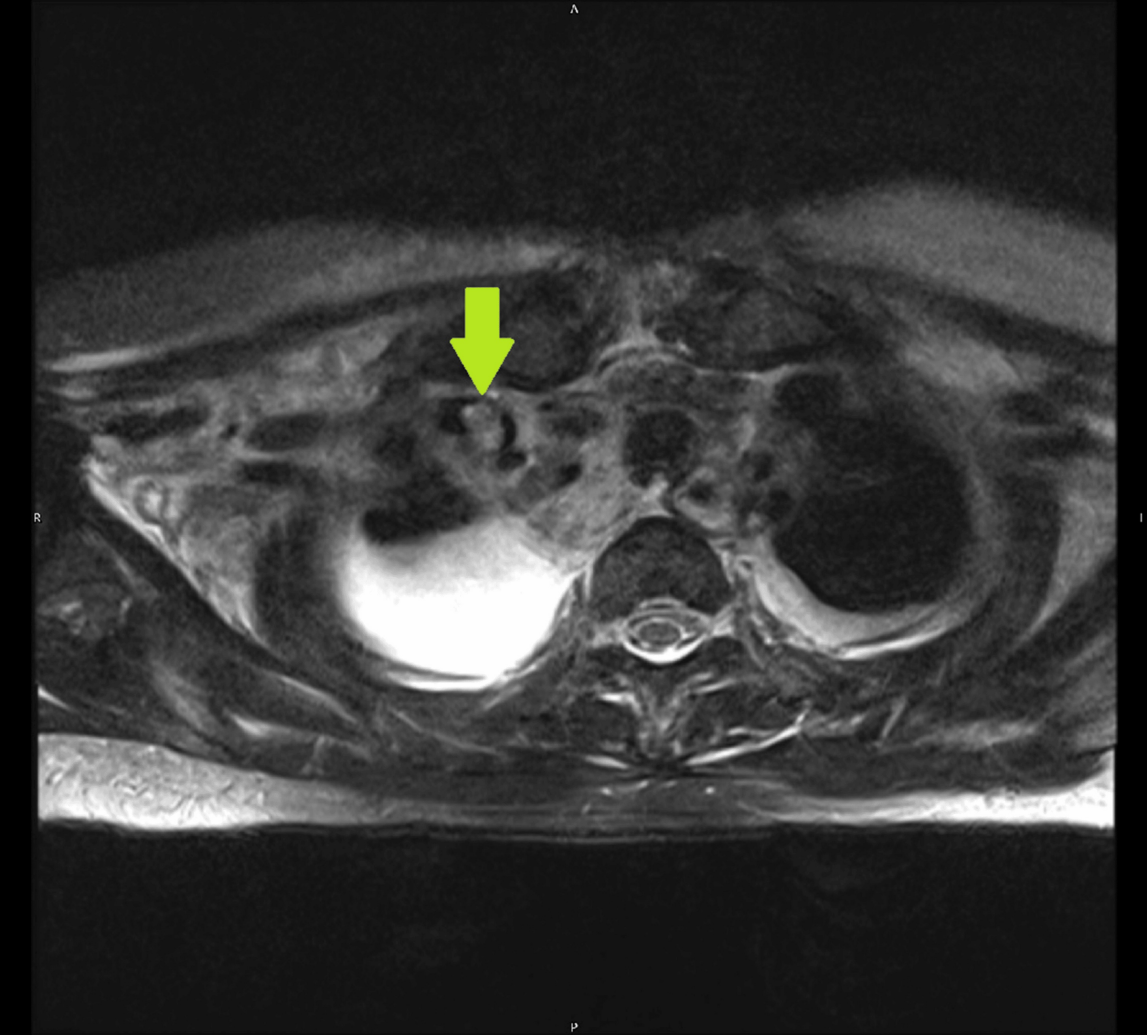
MRI T2 axial image showing thrombus at confluence of right internal jugular vein (IJV) and subclavian vein (arrow).
